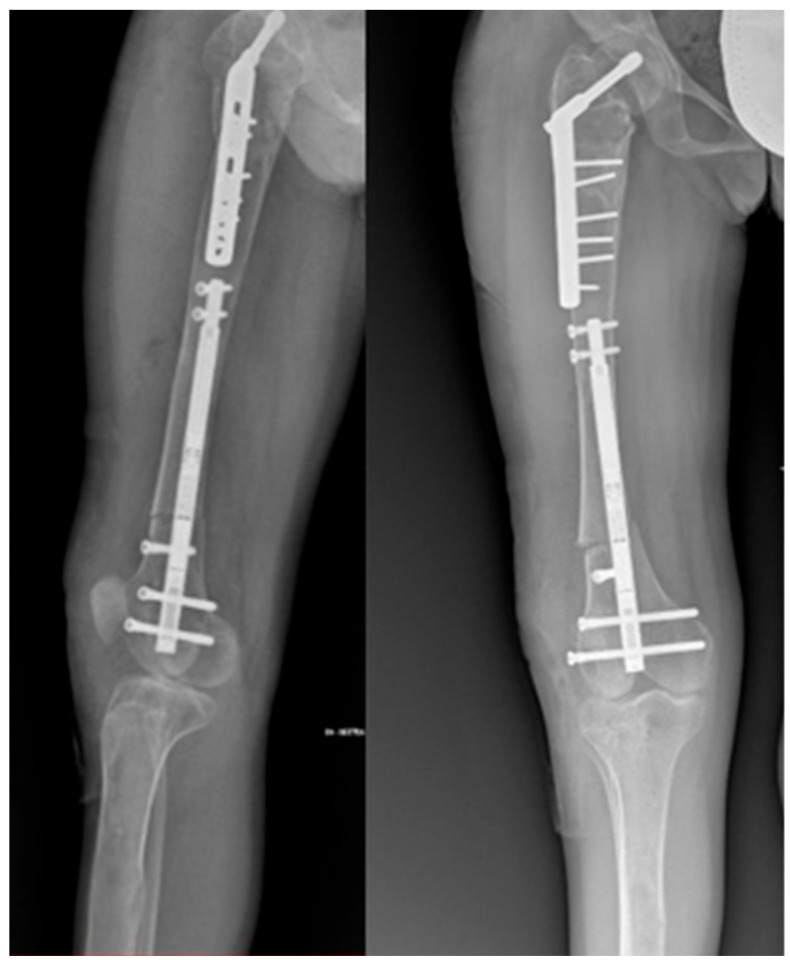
Postoperative X-ray with a retrograde magnetic intramedullary nail and a poller screw.
